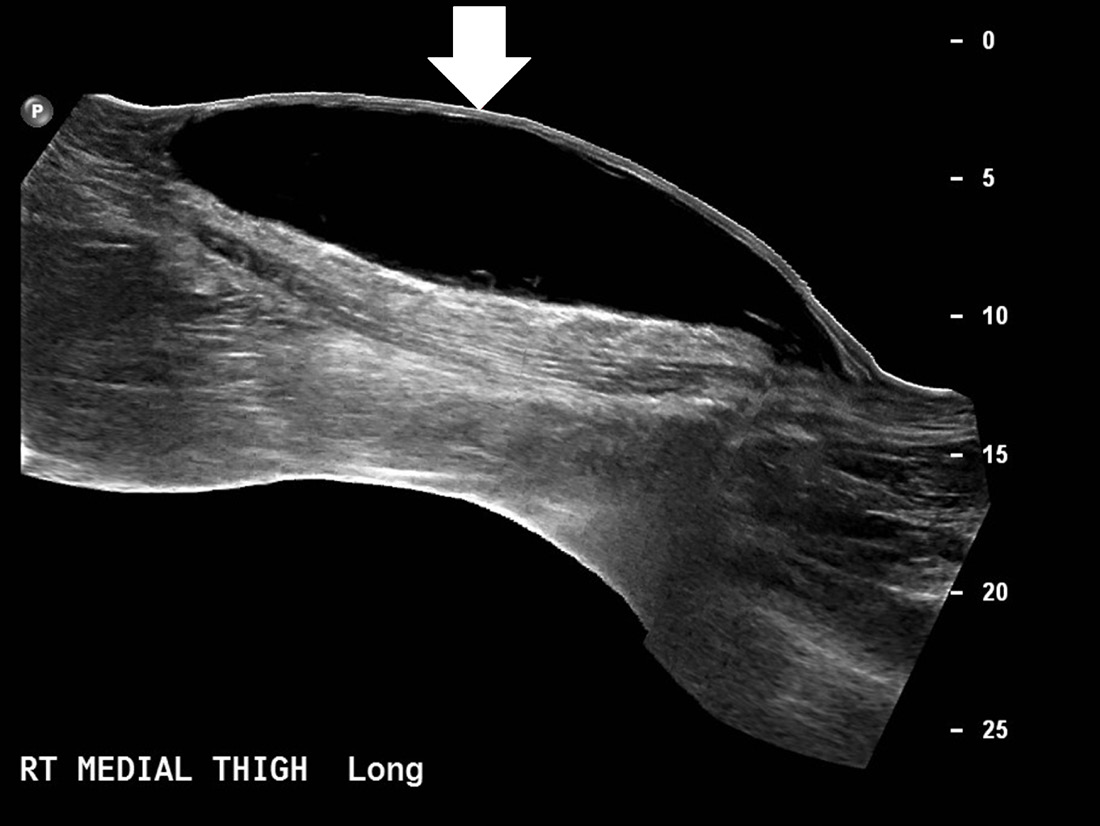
Morel-Lavallee lesion sonography with extended field of view along the long axis of the lesion shows fusiform shape and anechoic texture.
